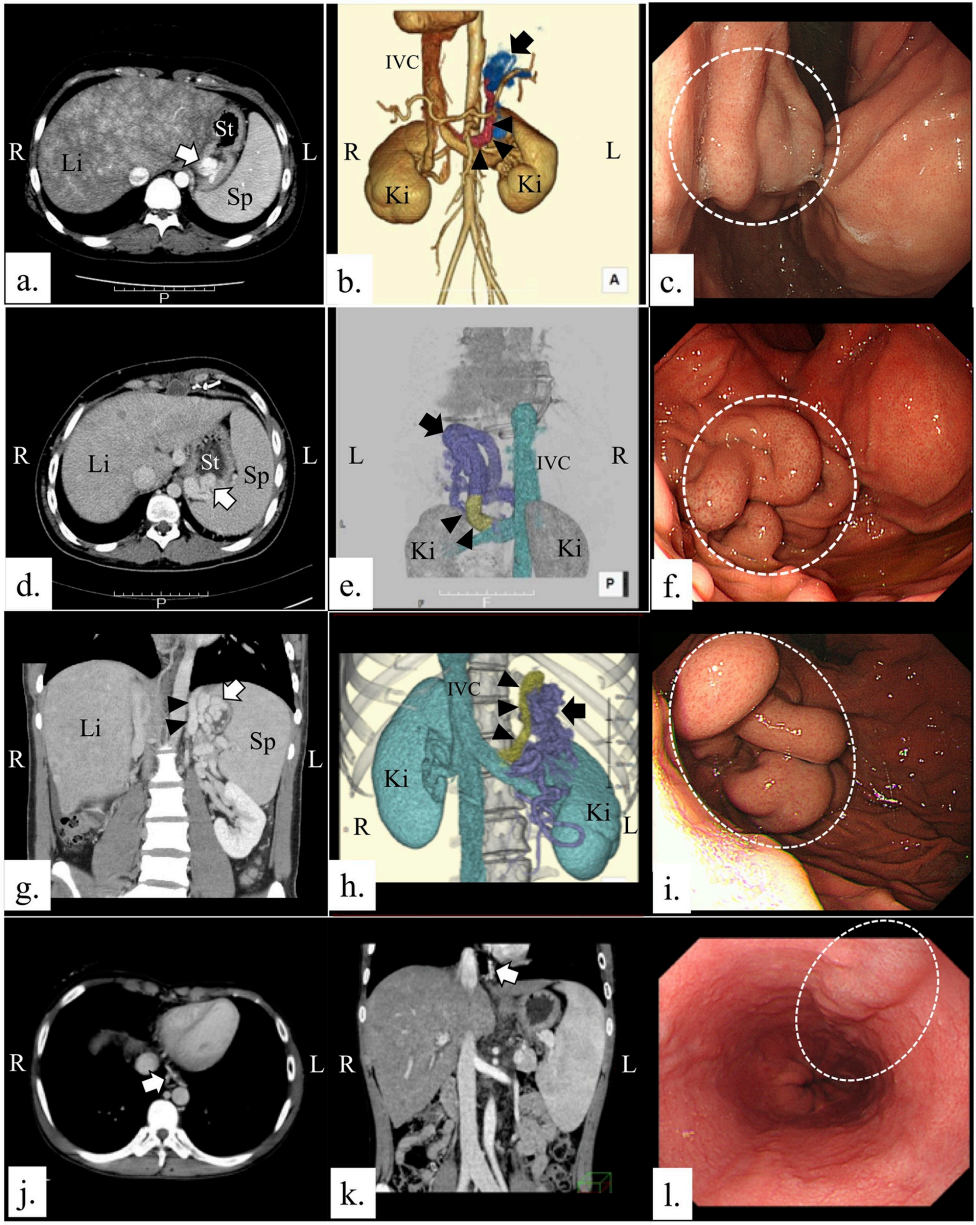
CT and endoscopic features of gastroesophageal varices in patients who underwent a Fontan procedure. (a, b) Abdominal dynamic CT images of a 19-year-old woman at 17 years after her Fontan procedure. (a) A portal phase image reveals a gastric varix (white arrow). (b) Three-dimensional CT image (anterior view) shows a gastric varix (blue, indicated by black arrow) draining into a gastrorenal shunt (red, indicated by black arrowheads). (c) Endoscopic image from the patient shown in Fig 2a and 2b. Esophagogastroduodenoscopy (EGD) reveals an enlarged nodular gastric varix (Lg-cf, F2, CW, RC0; indicated by dotted white circle). (d, e) Abdominal dynamic CT images of an 18-year-old man 14 years after his Fontan procedure. (d) Portal phase image reveals a gastric varix (indicated by white arrow). (e) Three-dimensional CT image (posterior view) shows a tortuous gastric varix (purple, indicated by black arrow) that drains into a gastrorenal shunt (yellow, indicated by black arrowheads). (f) Endoscopic image from the patient shown in Fig 2d and 2e. EGD reveals an enlarged nodular gastric varix (Lg-cf, F2, CW, RC0; indicated by dotted white circle). (g, h) Abdominal dynamic CT images of a 19-year-old man 13 years after a Fontan procedure. (g) Portal phase image reveals a gastric varix (indicated by white arrow) that drains into a gastrorenal shunt (indicated by black arrowheads). (h) Three-dimensional CT image (anterior view) shows a gastric varix (blue, indicated by black arrow) that drains into a gastrorenal shunt (red, indicated by black arrowheads). (i) Endoscopic image from the patient shown in Fig 2g and 2h. EGD reveals an enlarged nodular gastric fundal varix (Lg-f, F2, Cb, RC0) (indicated by dotted white oval). (j, k) Abdominal dynamic CT images of a 14-year-old boy 12 years after his Fontan procedure. The sagittal view (j) and coronal view (k) at the portal phase reveal a small esophageal varix (indicated by white arrows). (l) Endoscopic image of the patient shown in Fig 2j and 2k. EGD reveals a straight, small-caliber esophageal varix in the lower esophagus (Li, F1, Cw, RC0) (indicated by dotted white oval). GEV, gastroesophageal varix; IVC, inferior vena cava; Ki, kidney; L, left; Li, liver; R, right; Sp, spleen; St, stomach.
